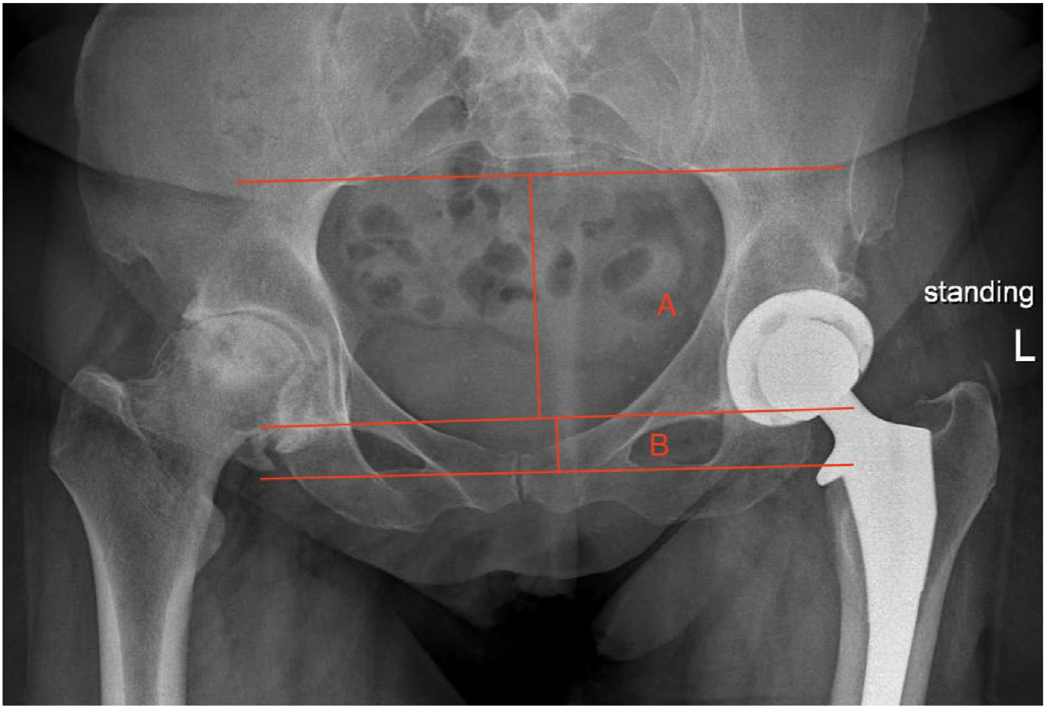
Postoperative AP plain film. Value A: Distance from the base of the sacroiliac joint to the superior margin of the obturator foramen. Value B: Height of the obturator foramen. Preoperative pelvic tilt was measured using the formula from Schwarz et al [18]: Tilt = −(ln[(B/A) × (1/0.483)]) / 0.051.
